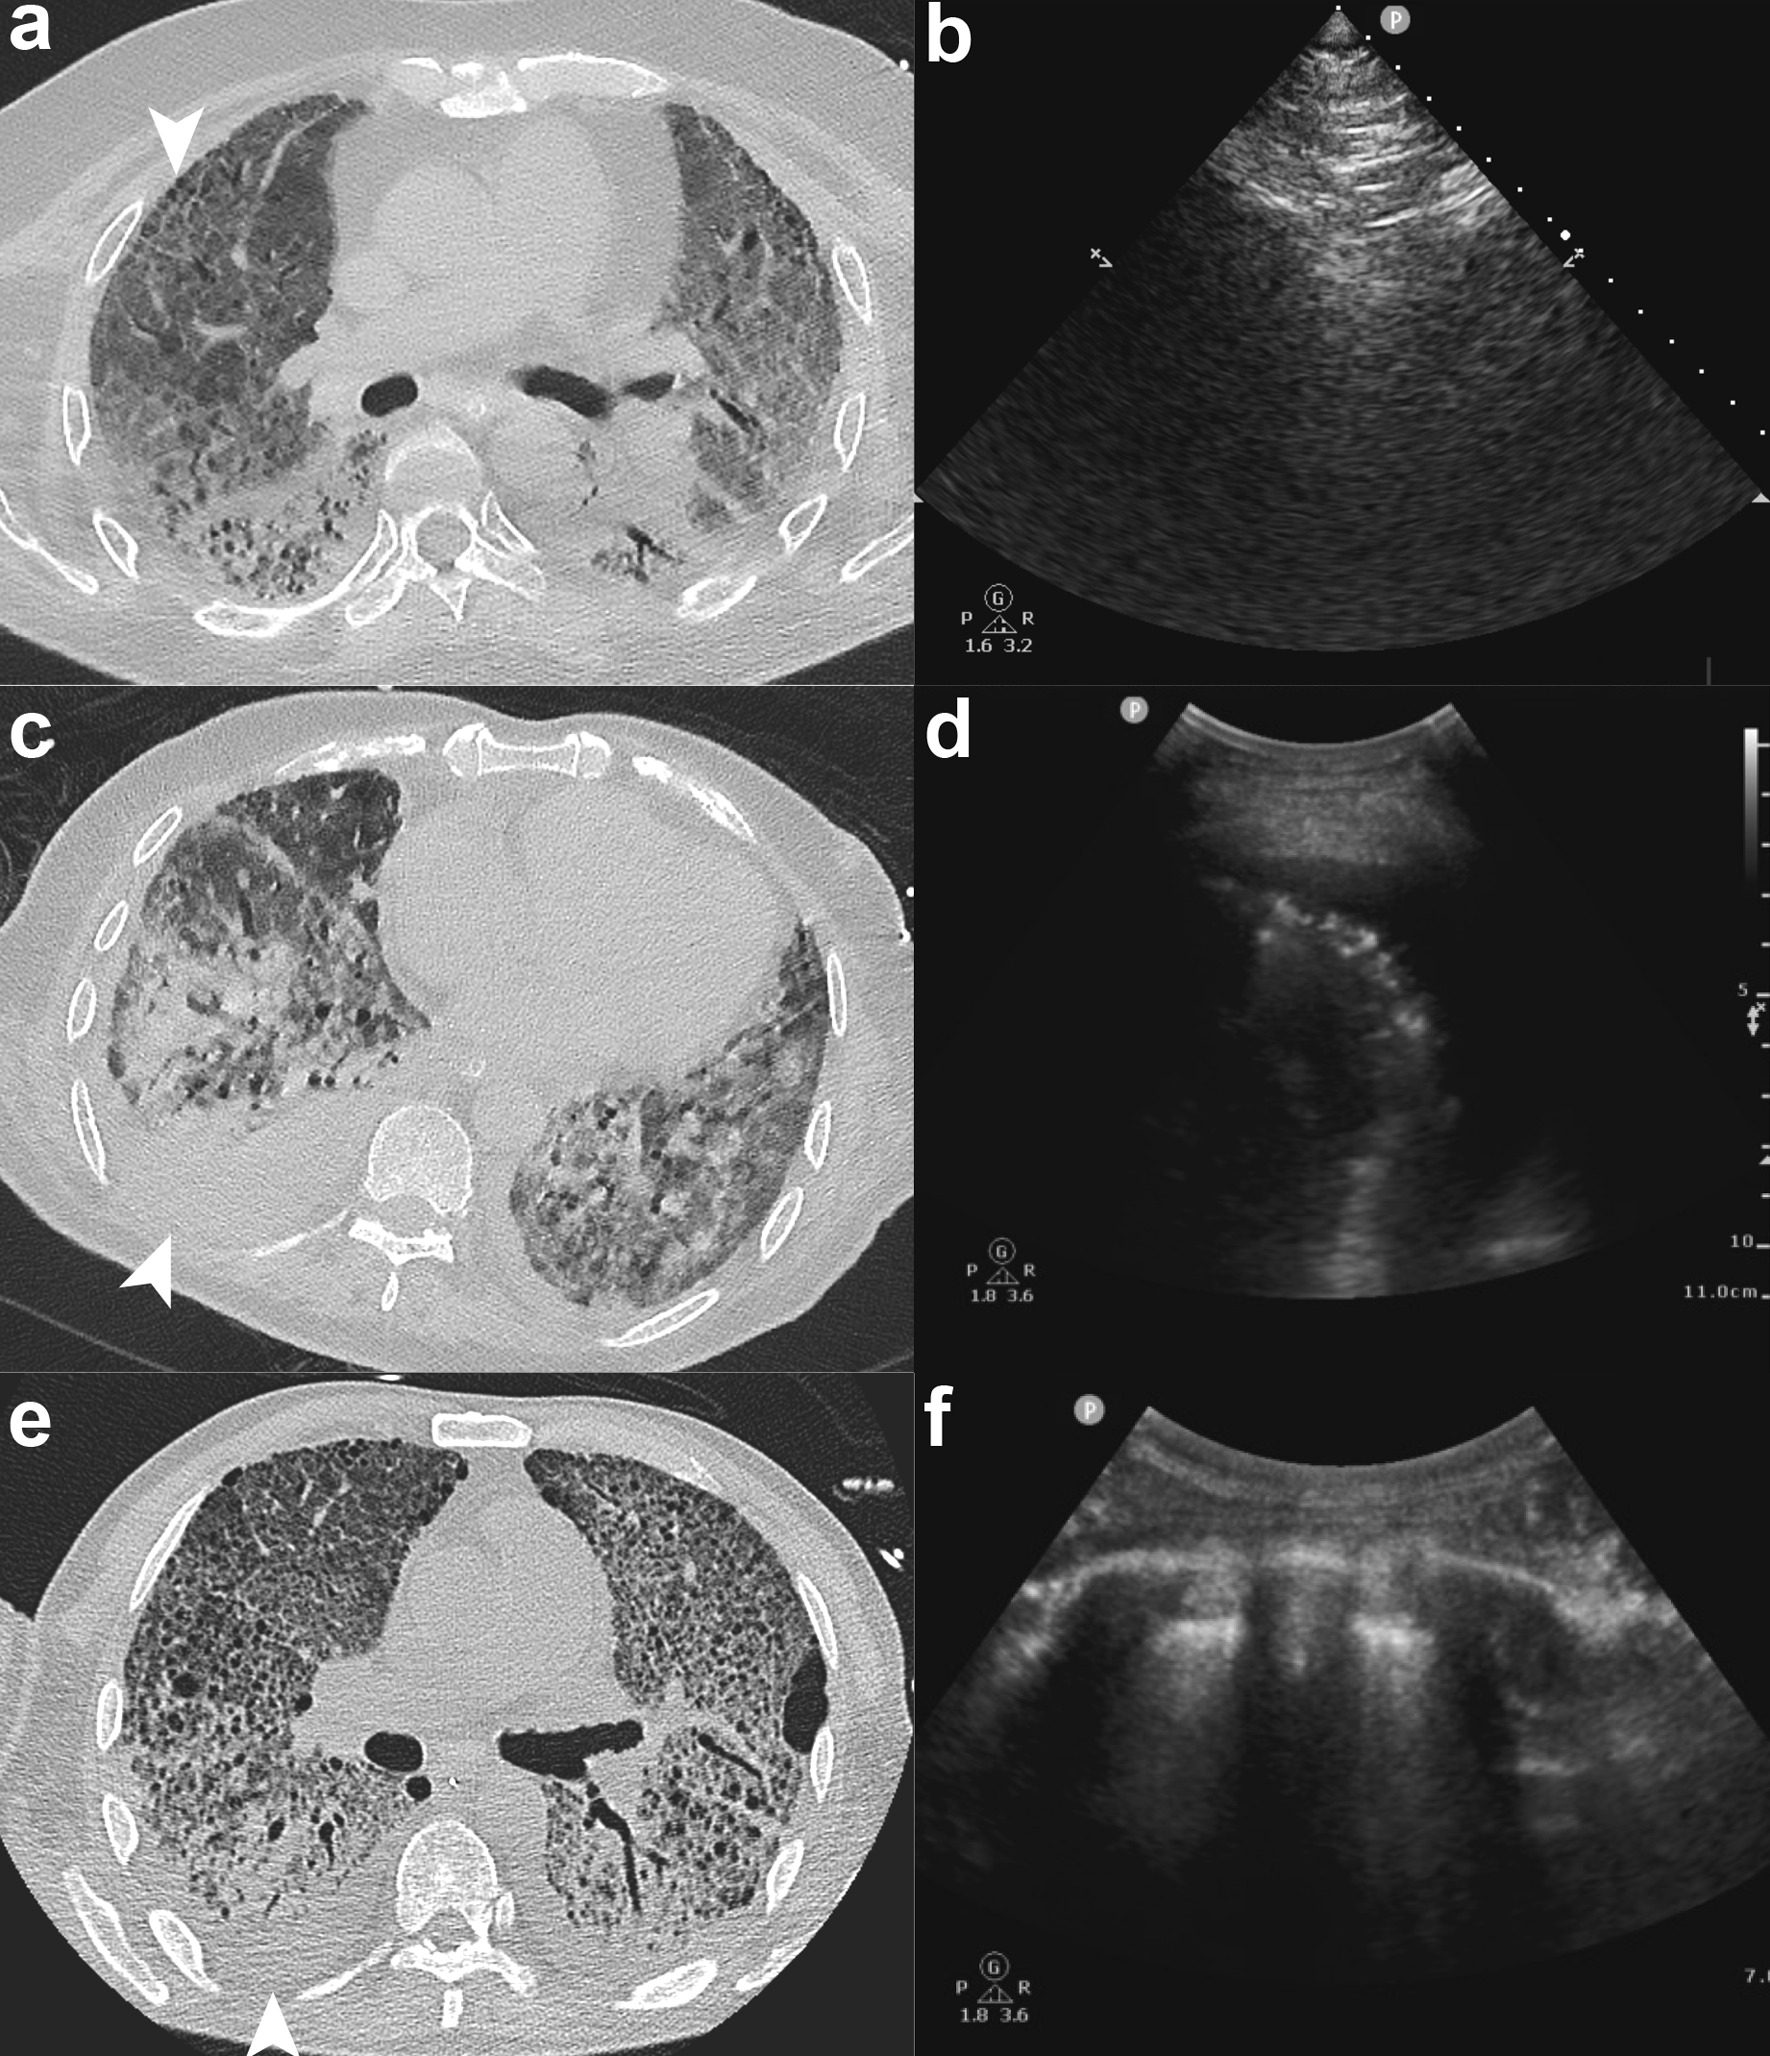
Biopsy sites for patient 2, 3 and 4. a, b Needle biopsy site was carefully chosen based on CT evaluation before procedure and sonography during the procedure. Right anterior segment was chosen for biopsy in patient 2. c, d And right posterior basal segment was chosen in patient 3. e For patient 4, right dorsal segment was chosen for puncture. f After closed thoracic drainage, sonography showed no air left in thoracic cavity
